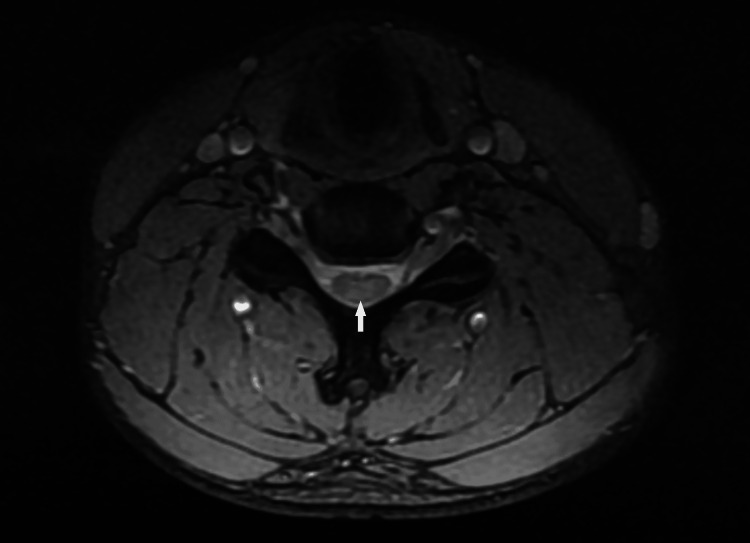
T2-weighted spoiled gradient echo (MERGE) transverse MRI of the cervical spine revealing hyperintense lesions in the dorsal columns of the spinal cord.
